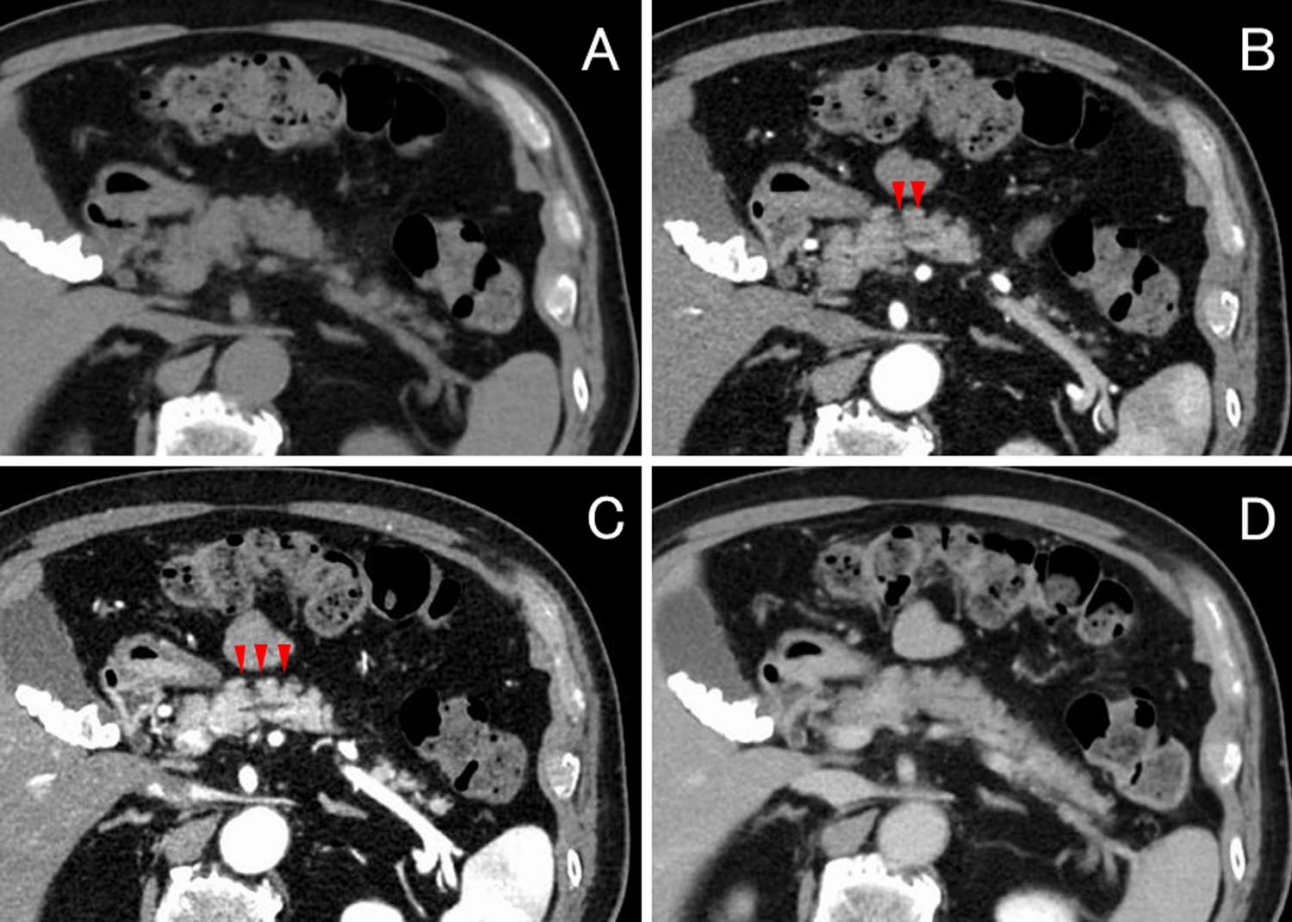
Contrast-enhanced computed tomography of the pancreas in four phases: A Unenhanced phase, B Arterial phase, C Portal venous phase, and D Equilibrium phase. The images show mild dilation of the main pancreatic duct in the body of the pancreas (red arrows), without any detectable mass lesion
